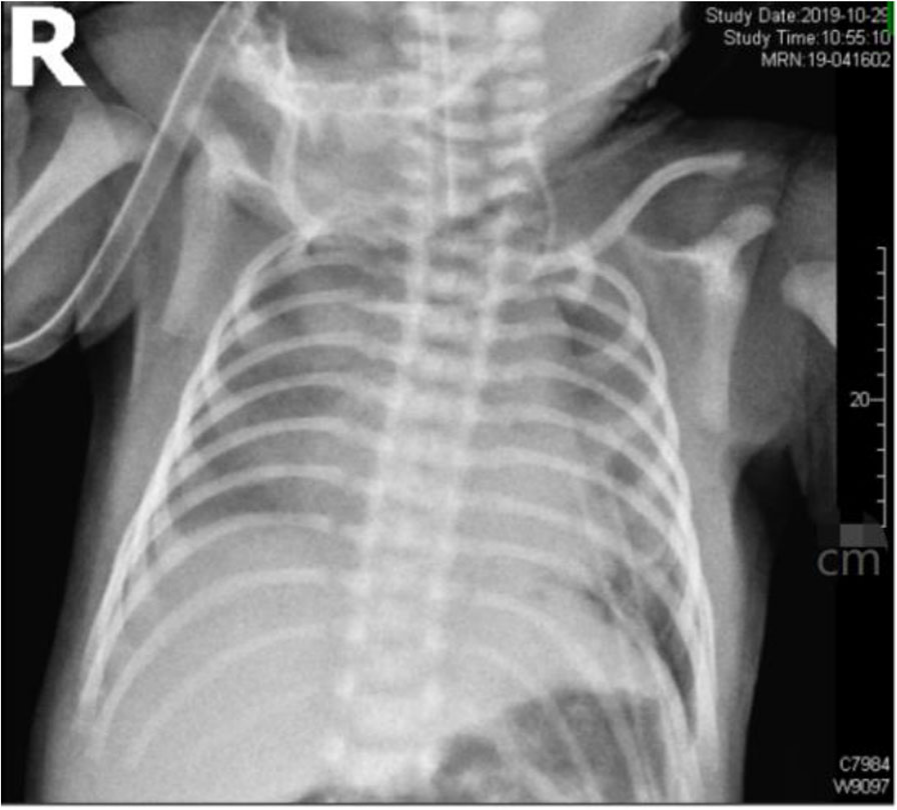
X-ray showing that the left lung recovered satisfactorily
